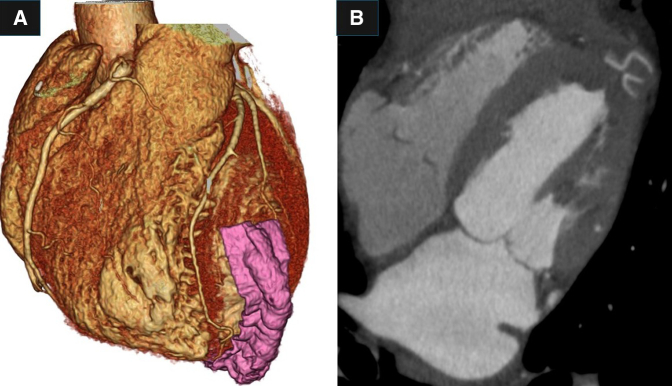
A, Three-dimensional reconstruction of postoperative computed tomography scan. B, Thin-slice image of postoperative computed tomography scan.
